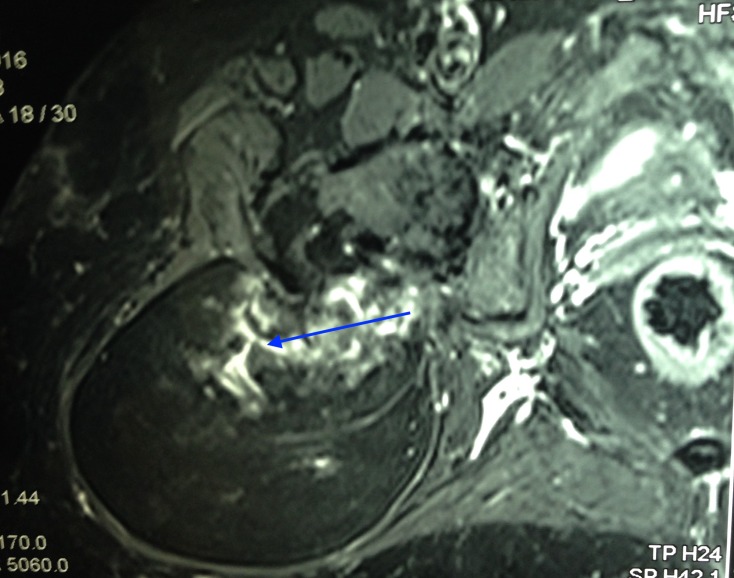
T2-weighted MRI showing tumour mass
